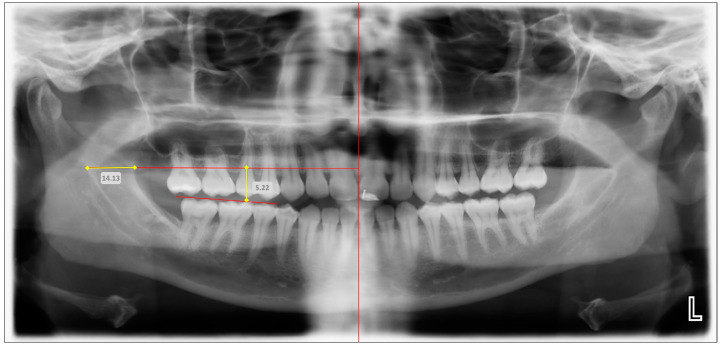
Representative image showing the digital measurements of the anteroposterior and superoinferior positions of the mandibular foramen (MF) (in mm) in an orthopantomogram (OPG). Red vertical line—true vertical line (TVL); Superior red horizontal line—perpendicular line drawn from anteroinferior aspect of MF to TVL; Inferior red horizontal line—mandibular occlusal plane; Horizontal yellow line—anteroposterior position of MF (in mm); and Vertical yellow line—superoinferior position of MF (in mm).
